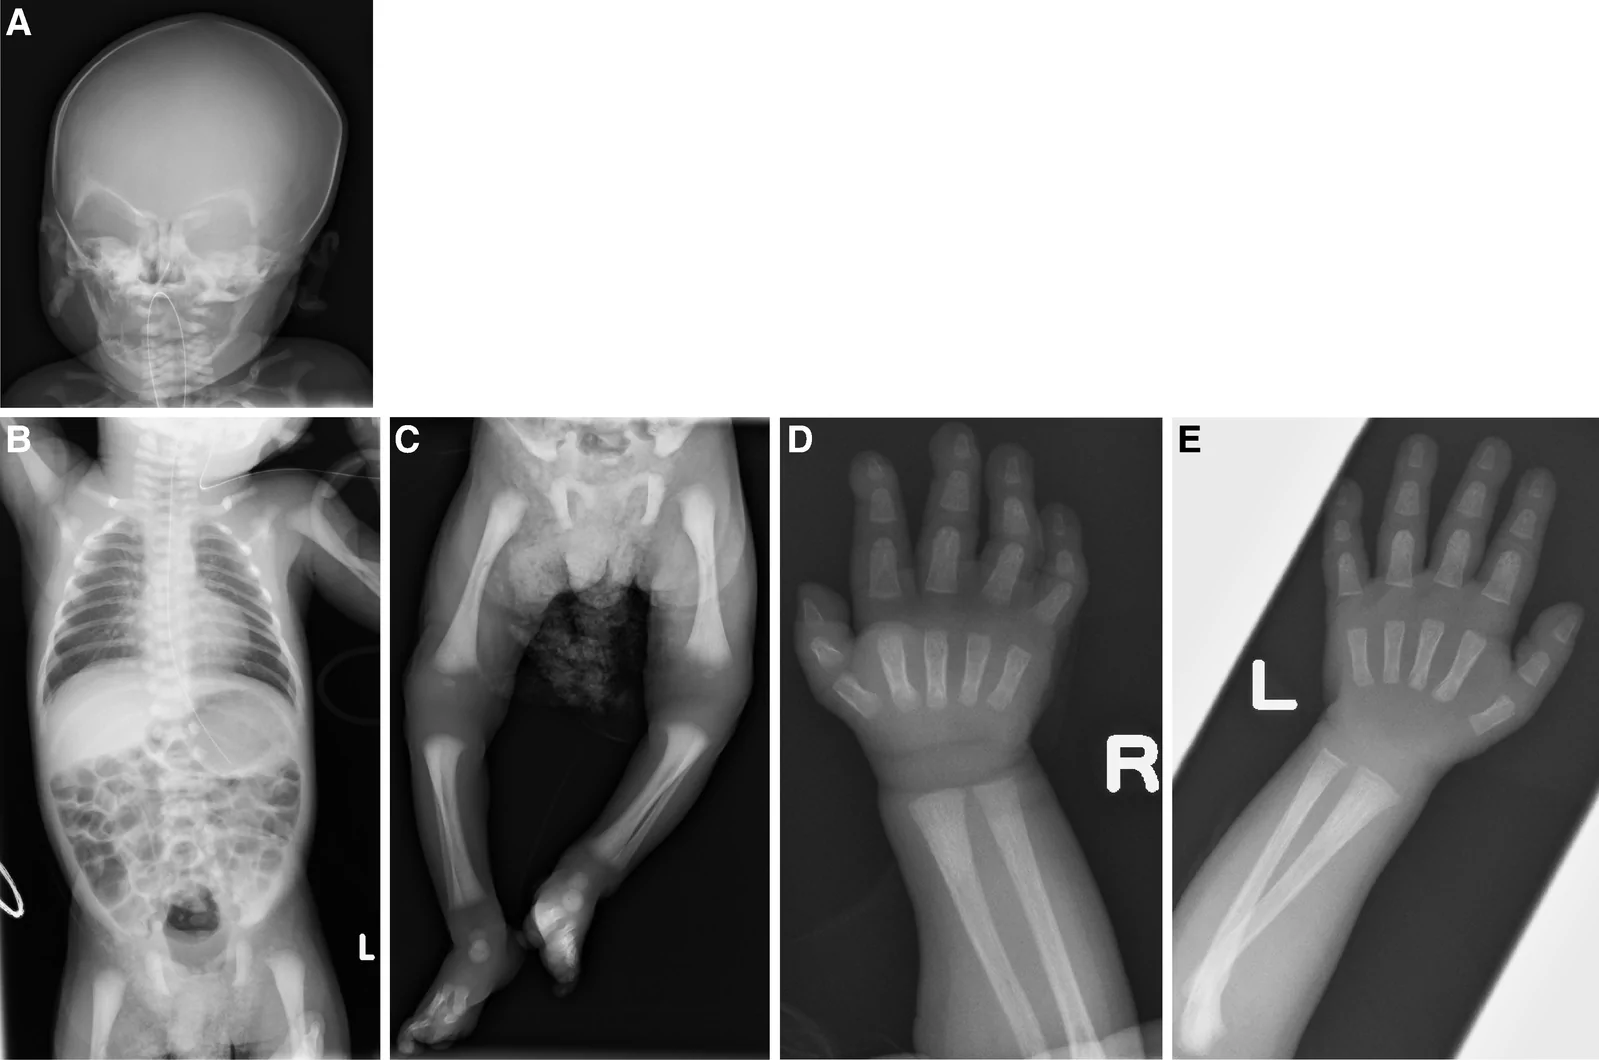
Radiographic findings of the infant. (A-C) Radiograph of the neonate on day 2. Despite extremely high serum FGF23 levels, there is no evidence of rickets or skeletal dysplasia. (D-E) Radiograph of the infant's hands at 1 month of age, revealing normal mineralization.
